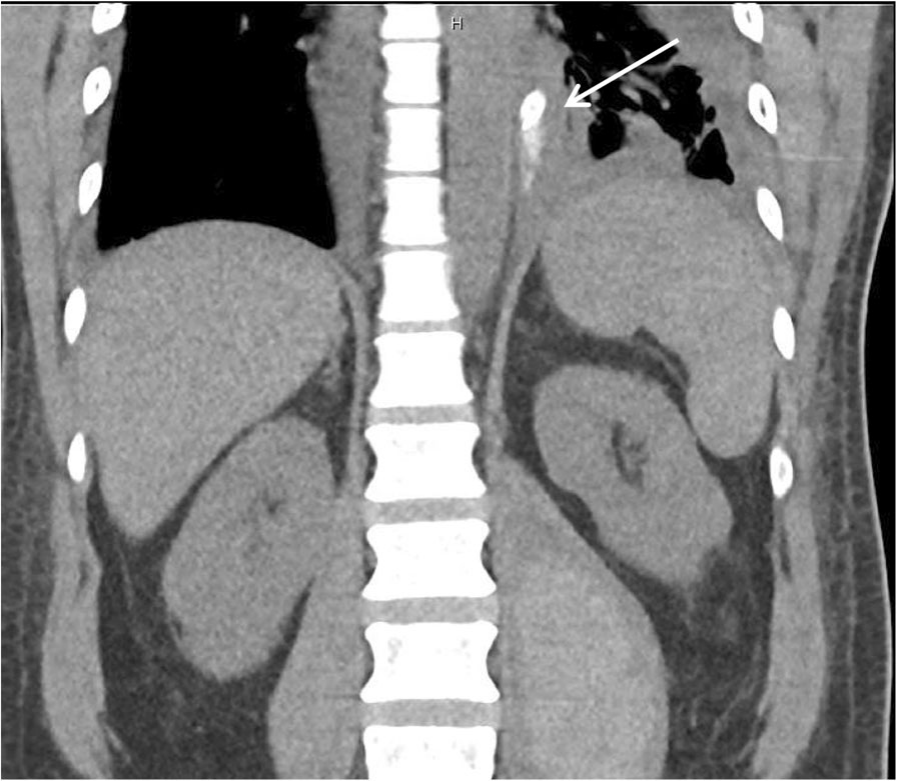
Computed tomography chest/upper abdomen repeated day 7 post-laparoscopy demonstrated extraluminal contrast extending into the left pleural space (arrow). The left pleural effusion has reduced in volume since insertion of intercostal catheter. Coronal view
